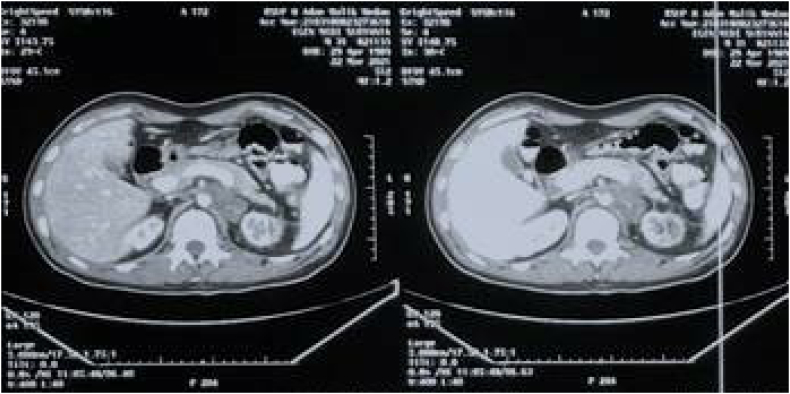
CT scan with contrast 3 months after BEP administration.
